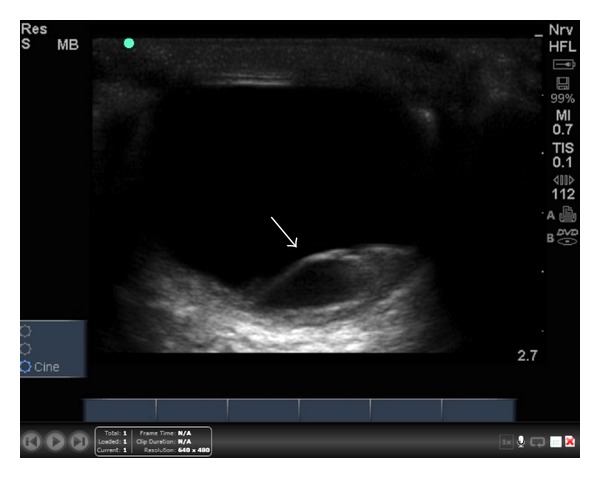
Left eye ultrasound shows retinal detachment.
